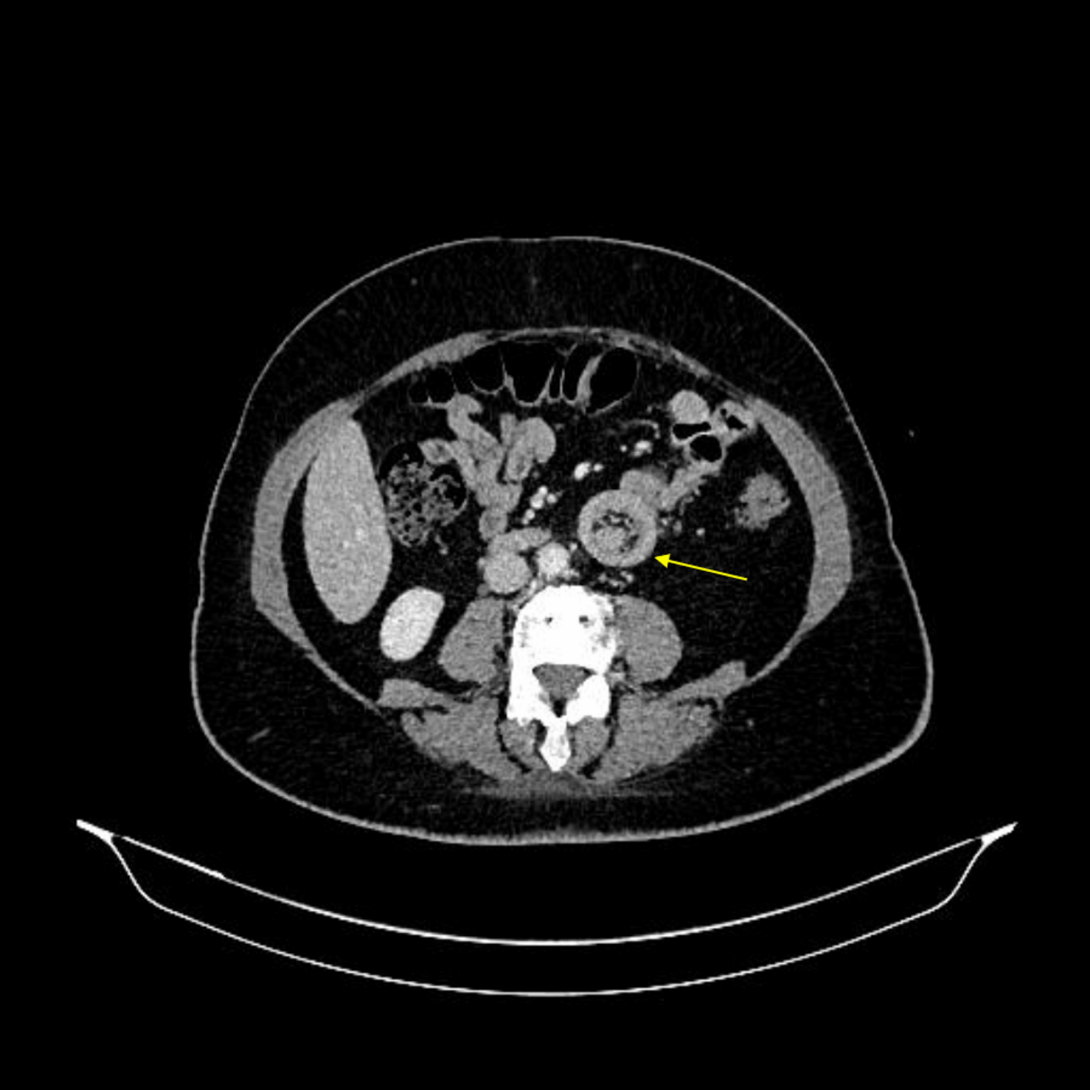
Abdominal CT scan image. The arrow indicates the location of the intussusception.
